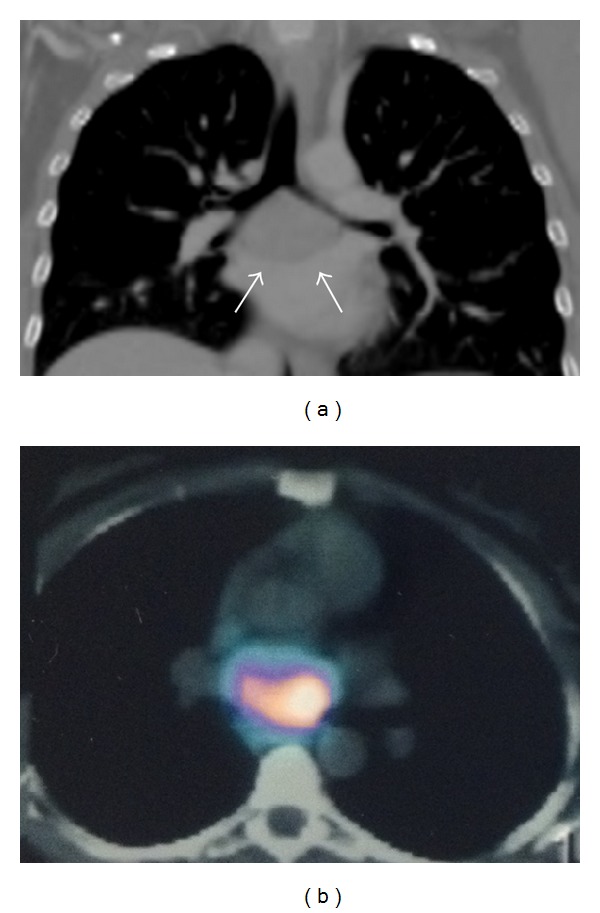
(a) Coronal view of chest computed tomography, revealing an intrapericardial 55 mm mass located at the roof of the left atrium (arrows). (b) ^123^I-metaiodobenzylguanidine chest scan showing high uptake by the intrapericardial mass.
